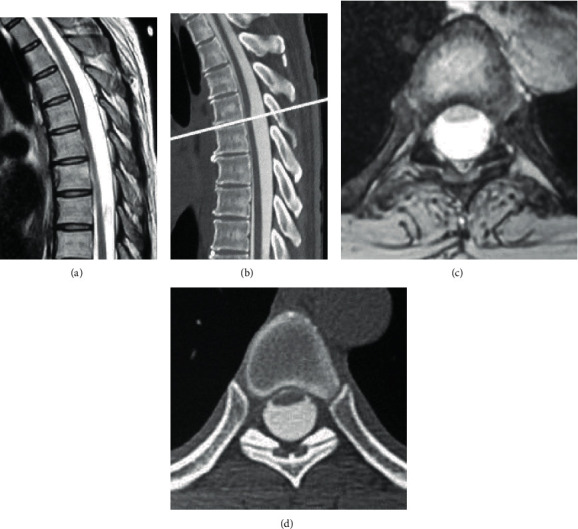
Preoperative T2-weighted MRI and CT myelography. (a, b) Sagittal MRI and CT myelography show the “scalpel sign.” The white line indicates the T5/T6 disc level. (c, d) Axial MRI and CT myelography show spinal cord deviation.
